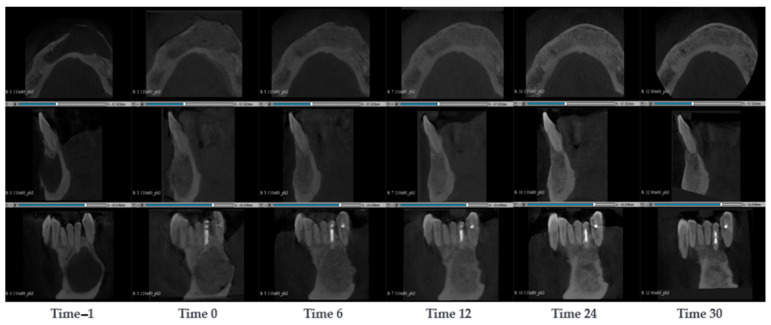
CBCT images of a 69-year-old male with a large mandibular cyst. The cyst was removed and SmartBone^®^ microchips were inserted. The images show the progression of bone healing over time, from pre-surgery (Time −1) to 30 months post-surgery (Time 30).
